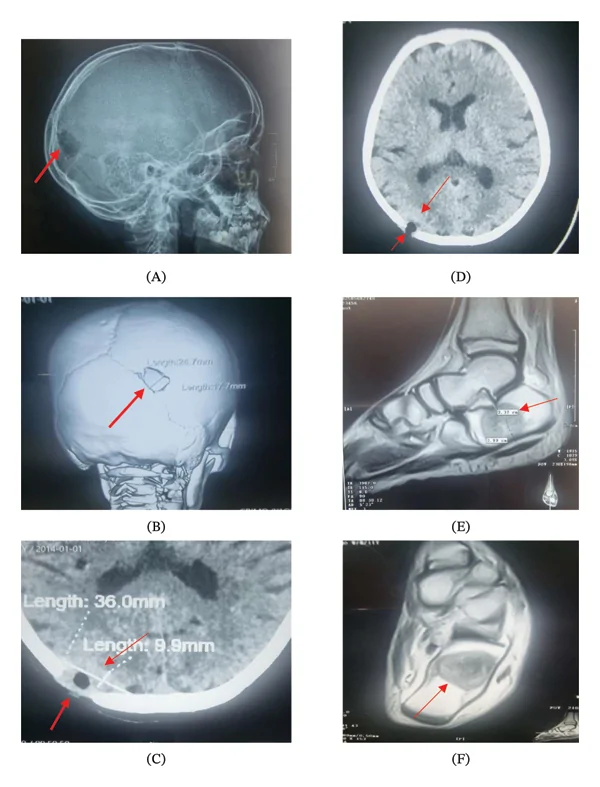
(A) Skull X‐ray (lateral view) osteolytic lesion around the parietal zone. (B) Computed tomography of the skull, destructive bone lesion of the posterior right parietal. (C) CT scan of the head with contrast on parenchyma windows showing right osteolytic parietal lesion with underlying epidural enhancement. (D) CT scan of the head with contrast on parenchyma windows showing right osteolytic parietal lesion with underlying epidural enhancement. (E) T1 sequence showing a hyperintense circular lesion in the left calcaneus. (F) T2/STIR weighted sequence (axial section) showing a hypointense lesion in the left calcaneus.
